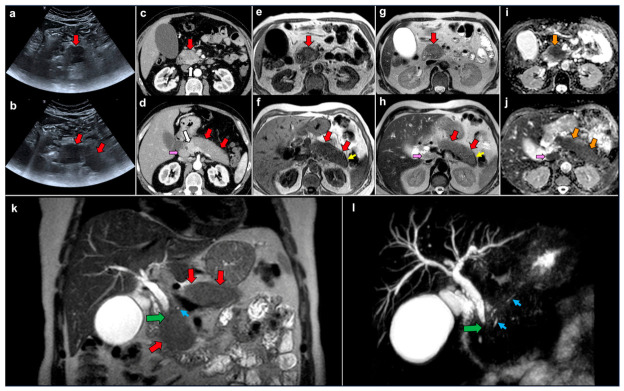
Multifocal AIP in a male patient presenting with epigastric pain and jaundice, showing elevated bilirubin, mildly increased serum amylase and raised serum IgG4 levels. (a,b) Conventional US shows a hypoechoic pseudomass in the pancreatic head (a, red arrow) and hypoechoic enlargement of the pancreatic body and tail (b, red arrows). (c,d) Pancreatic-phase CT demonstrates reduced enhancement of the pancreatic head, body and tail (red arrows), with sparing of normally enhancing parenchyma in the uncinate process and pancreatic neck (white arrows). Note also the presence of an enlarged inflammatory precaval lymph node ((d), pink arrow). (e,f) Axial T1WI shows hypointensity of the involved pancreatic segments (red arrows) and a hypointense capsule-like “halo” ((f), yellow arrow). (g,h) Axial T2WI demonstrates mildly increased signal of the affected segments (red arrows), with a hypointense capsule-like “halo” more evident in the pancreatic tail ((h), yellow arrow) and the precaval lymph node (pink arrow). (i,j) ADC maps show low values in the affected pancreatic segments (orange arrows) and the precaval lymph node (pink arrow). (k,l) Coronal T2WI and 3D MRCP with MIP reconstruction depict diffuse pancreatic swelling (red arrows), partial visualisation of the MPD (blue arrows) with long skipped strictures, and stenosis of the intrapancreatic CBD (green arrows) with upstream biliary dilatation.
